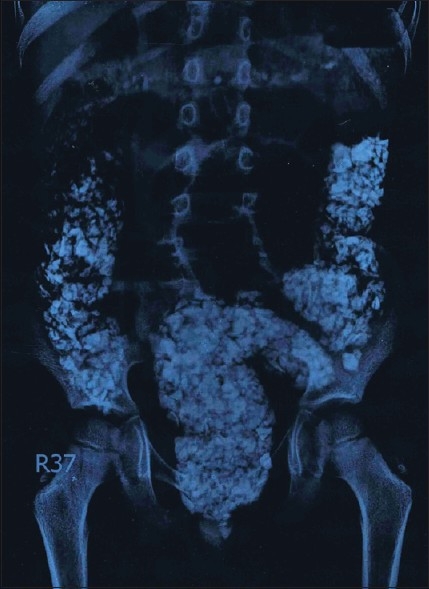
Abdominal X-ray at presentation
